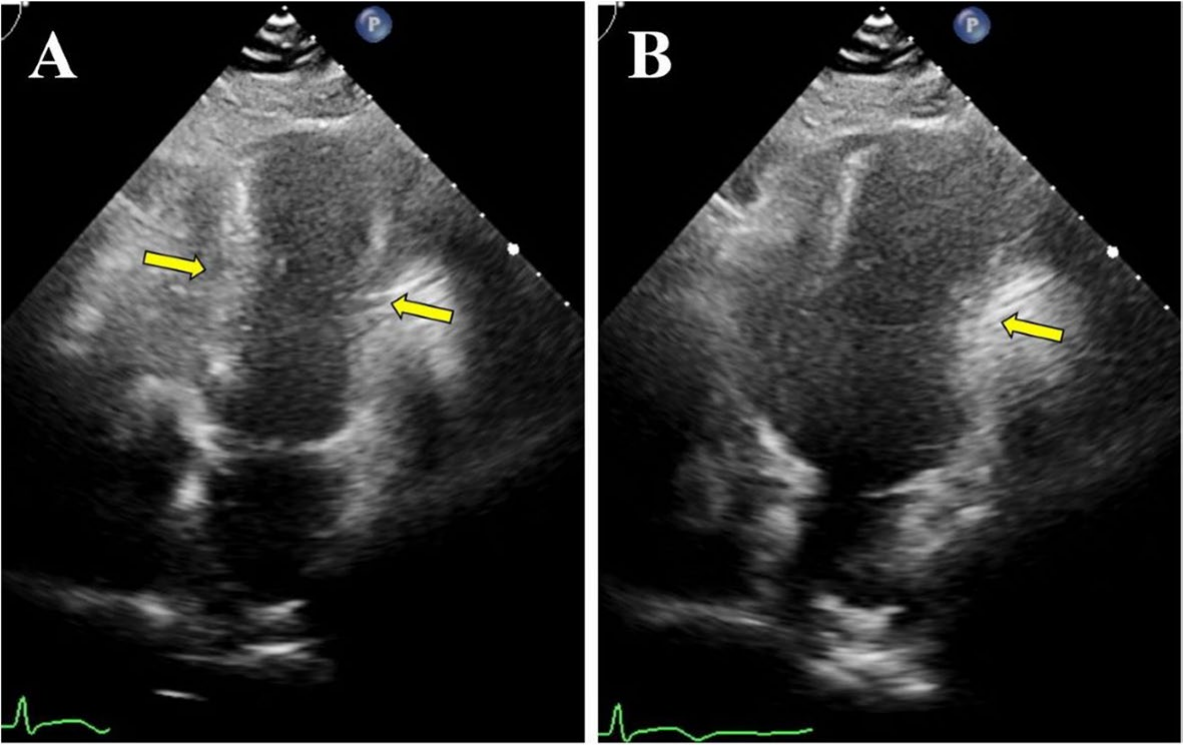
Transthoracic echocardiography. Apical four-chamber views in systole (A) and diastole (B) show both ventricles are compressed from the outer side (yellow arrow)
